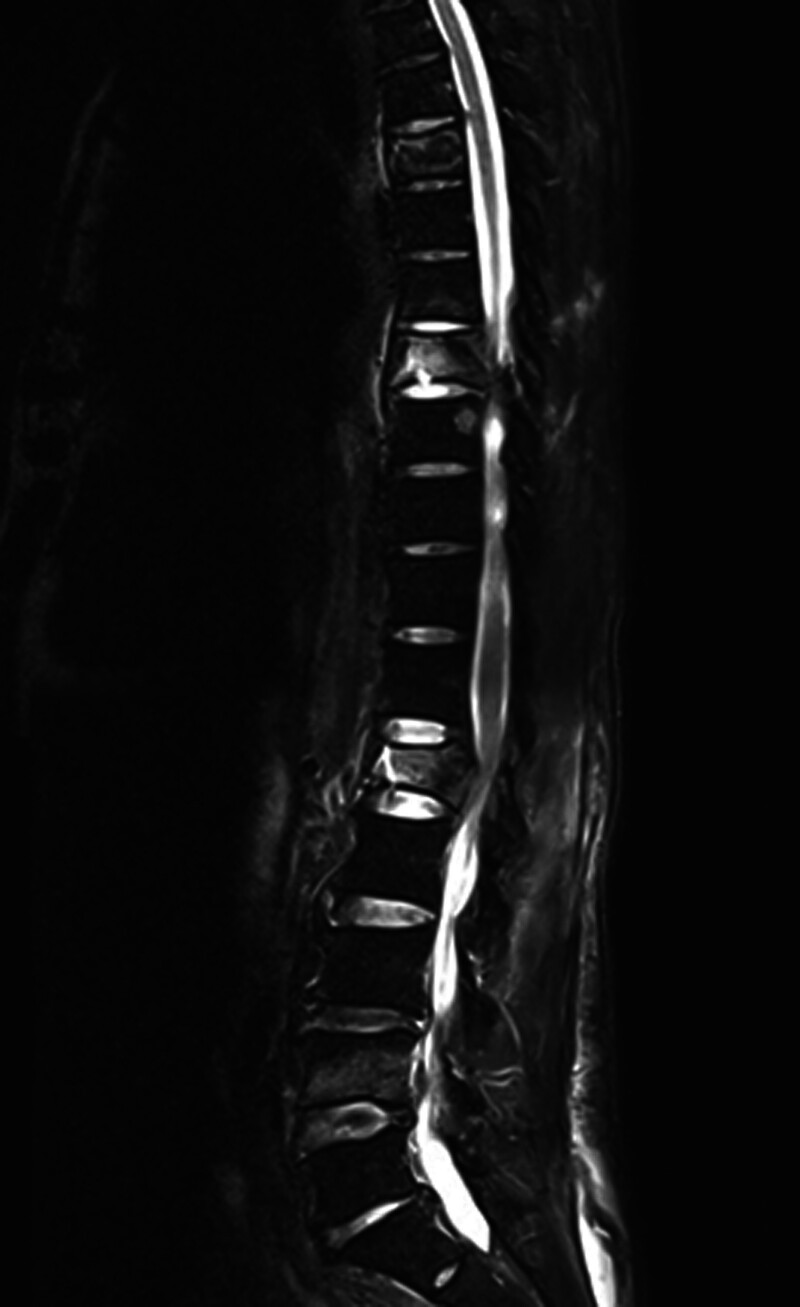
Sagittal T2-weighted magnetic resonance (MRI) images of the thoracic and lumbar spine. MRI = magnetic resonance imaging.
